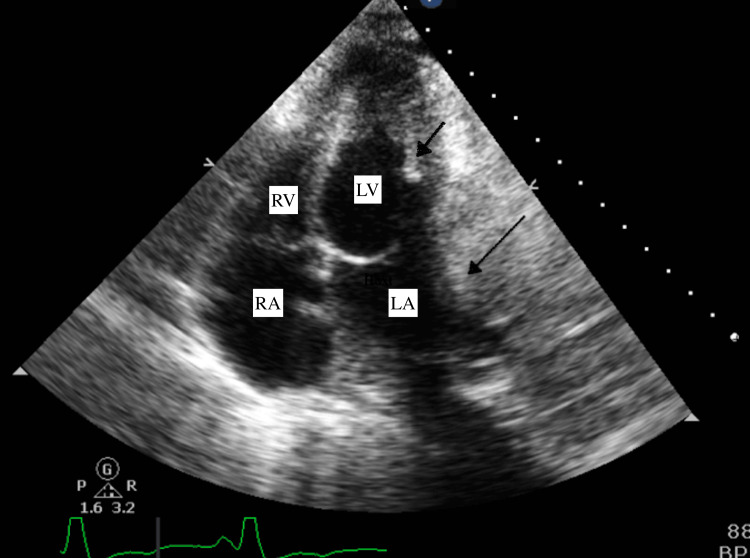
Echocardiographic apical 4 chamber view Showing the lung mass is pressing and invading the base of the left ventricle and left atrium (short and long black arrow respectively).
